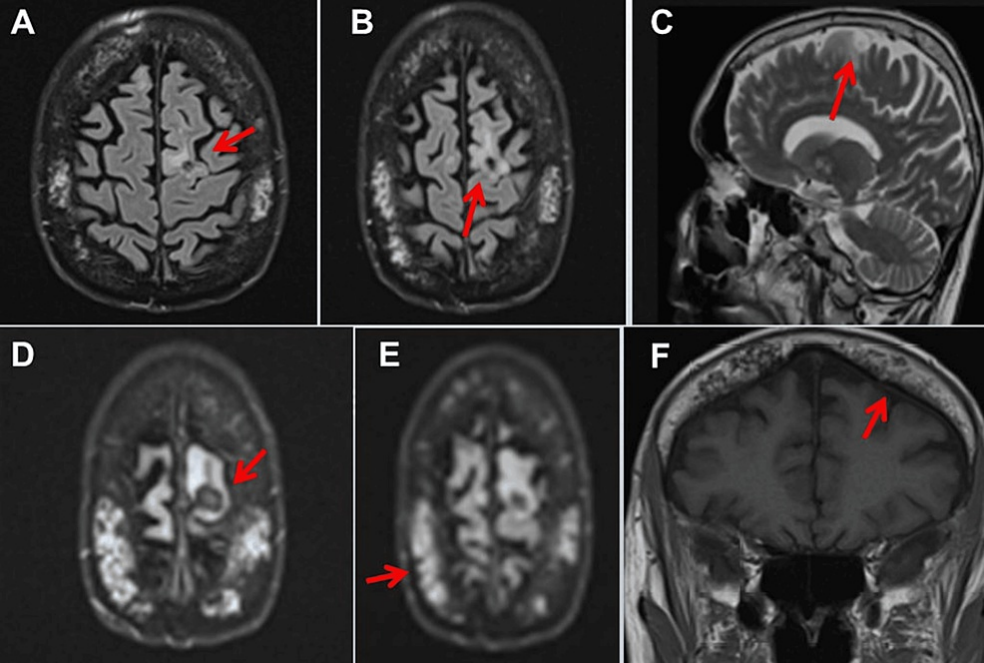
Cerebral MRI at age 63 shows a previously described right frontal mass lesion with perifocal edema and small edematous zones in a left parieto-occipital subcortical, right temporal, and bilateral frontal distribution (panels A to E). There is also evidence for leptomeningeal thickening, possibly secondary to previous meningeal carcinomatosis (panel F).
